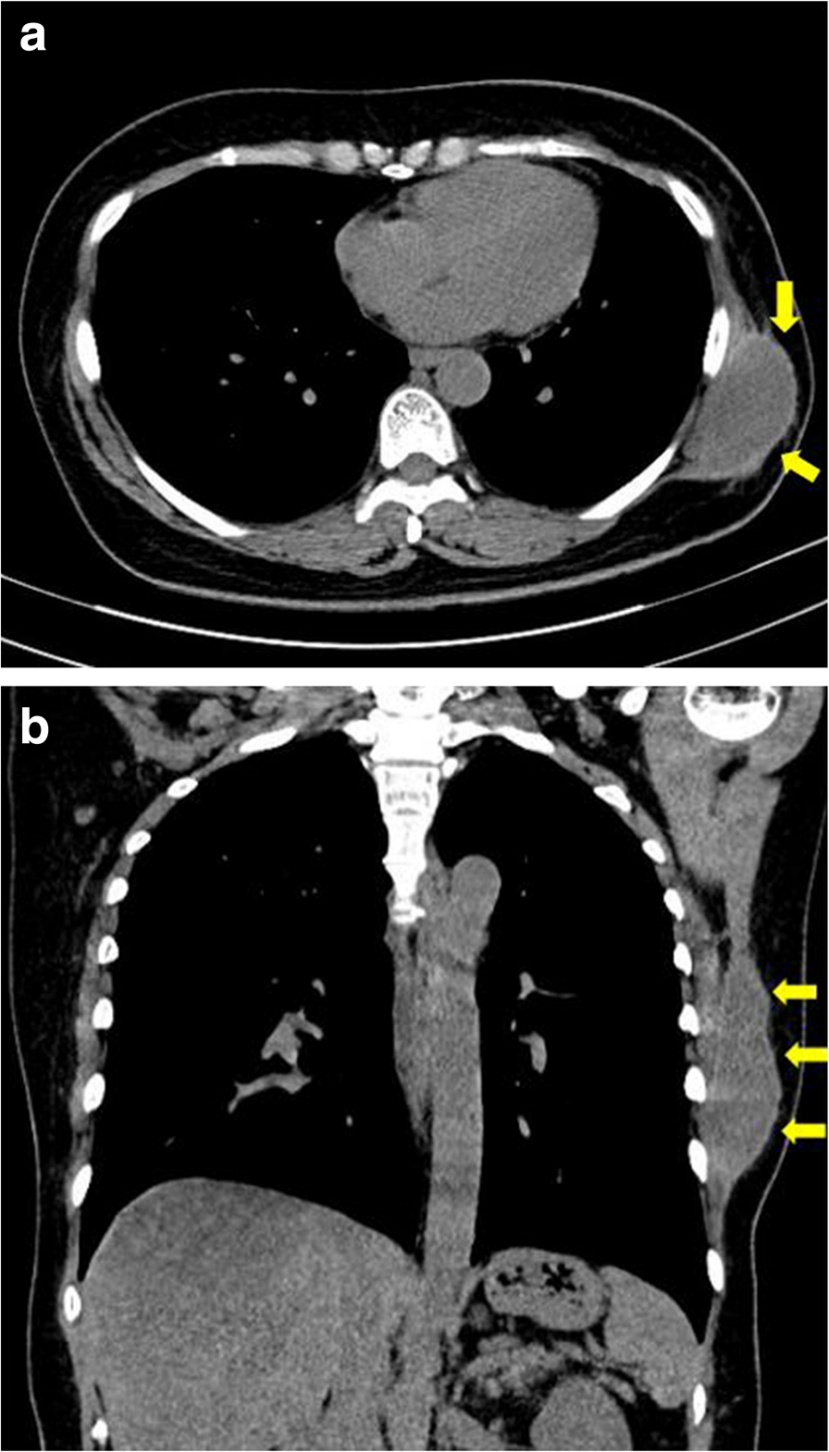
Preoperative computed tomography findings. Late solidified hematoma (yellow arrow) in the form of cystic mass (4 × 5 cm in size) is observed in the left LD donor site. (a) axial view, (b) coronal view
